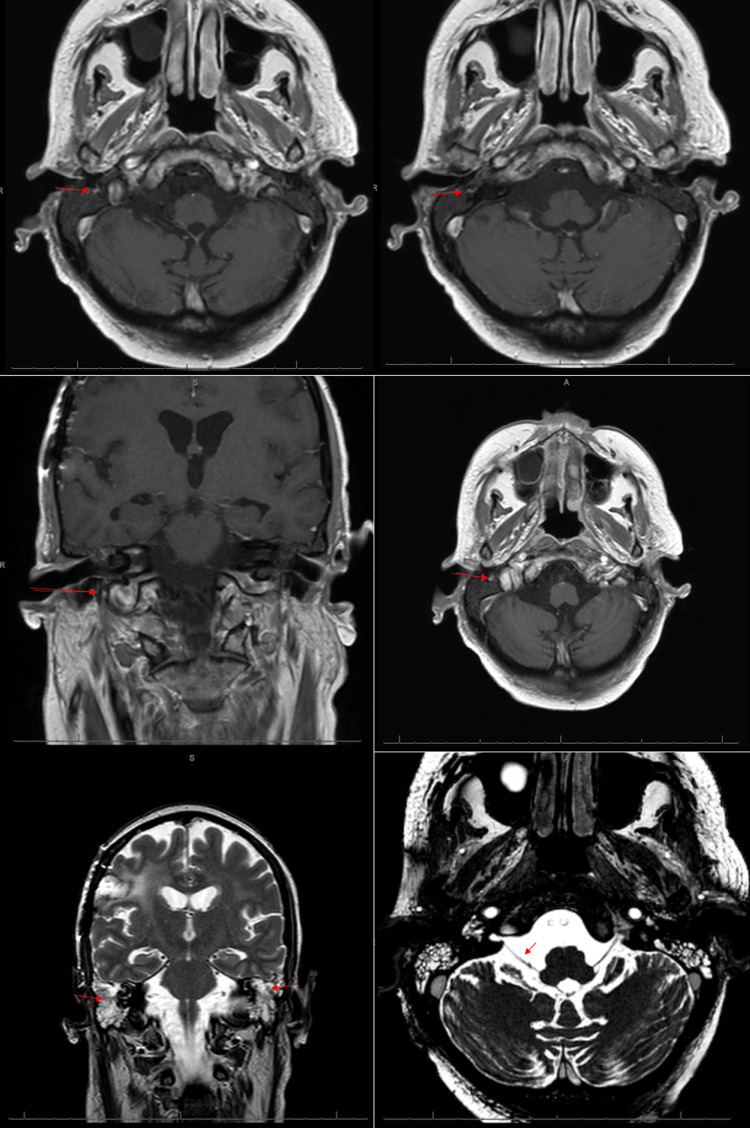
MRI brain with contrast Top Row: T1-weighted axial cross-sections demonstrating a focal area of high signal intensity seen adjacent to the right facial nerve. Middle Row: T1-weighted coronal and axial cross-sections demonstrating minimal enhancement of the descending intra-mastoid segment distally of the right facial nerve just proximal to the stylomastoid foramen. Bottom Row: T2-weighted coronal cross-section demonstrating right post-surgical encephalomalacia and opacification of the bilateral mastoid air cells (left). Axial cross-section demonstrating patency of cranial nerve VII exiting the pons (right).
